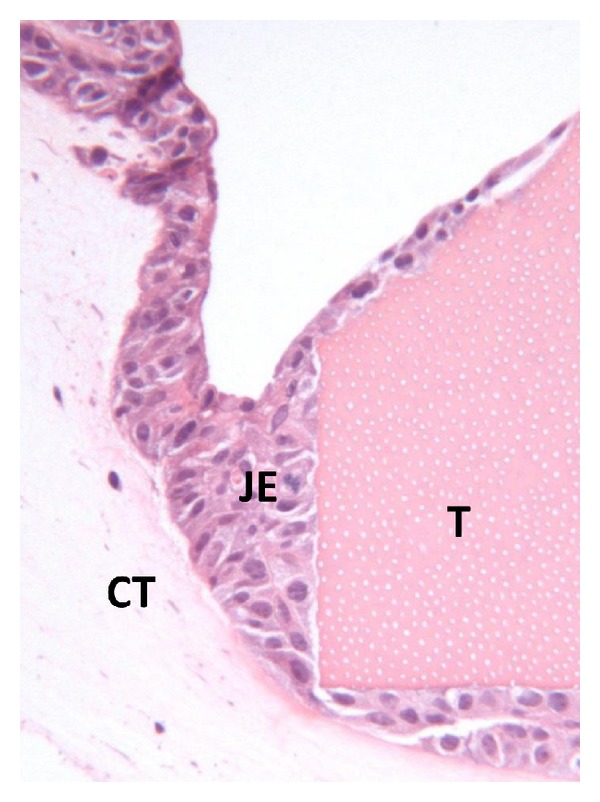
Organotypic culture model of the JE shows the structures of the dentogingival junction, JE = *in vitro* junctional epithelium, T = tooth, CT = connective tissue. When biofilm is placed on the top of the model host-bacteria interaction can be studied.
